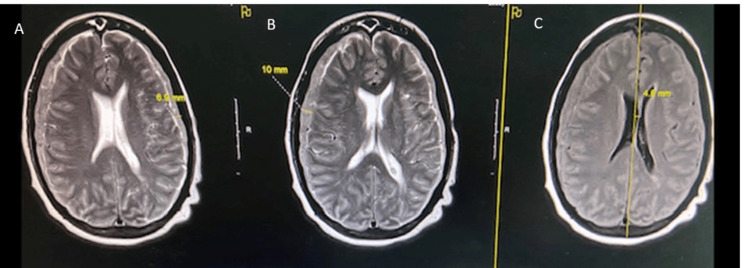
Figures A and B: Brain MRI of the T2 blade (axial view) showing increased signal within the bilateral temporal lobes as well as cortical thickening and edema. Figure C: T2 FLAIR (axial view), showing increased mass effect with a leftward midline shift of 5 mm.
